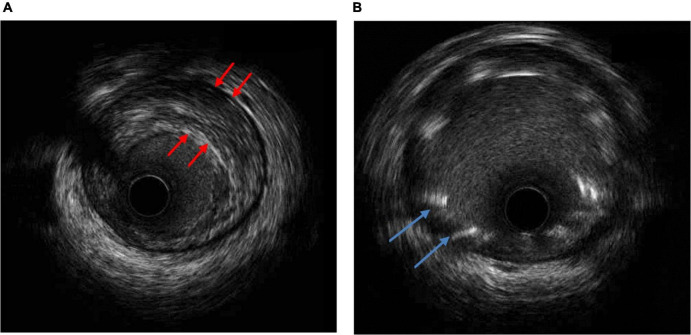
(A) Intravascular ultrasound (IVUS) of the narrowest lesion site showing a fibrotic plaque (red arrowhead). (B) IVUS post-stenting with fair stent expansion without stent edge dissection or stent malapposition (blue arrowhead).
